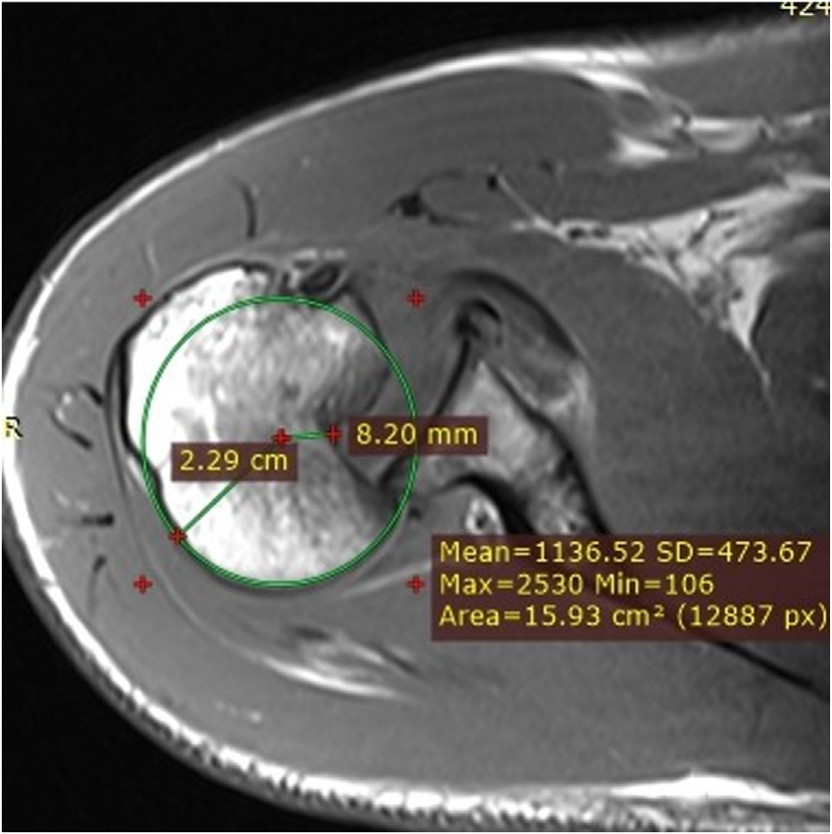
1st patient: MRI scan showing PSD, reverse Hill-Sachs lesion, impaction involving approximately 40% of the articular surface of the humeral head, and an intact posterior glenoid rim.
